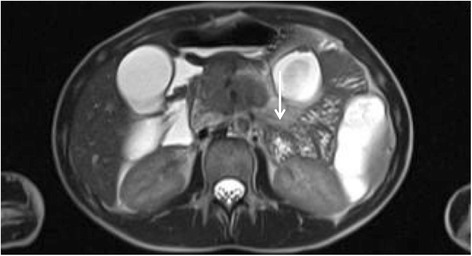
Axial, T2-weighted, half-Fourier single-shot turbo spin-echo hydromagnetic resonance imaging scan of the patient’s abdomen. Retroperitoneal tissue augmentation of the dorsal pancreas is visualized with contrast enhancement (*arrow*)
